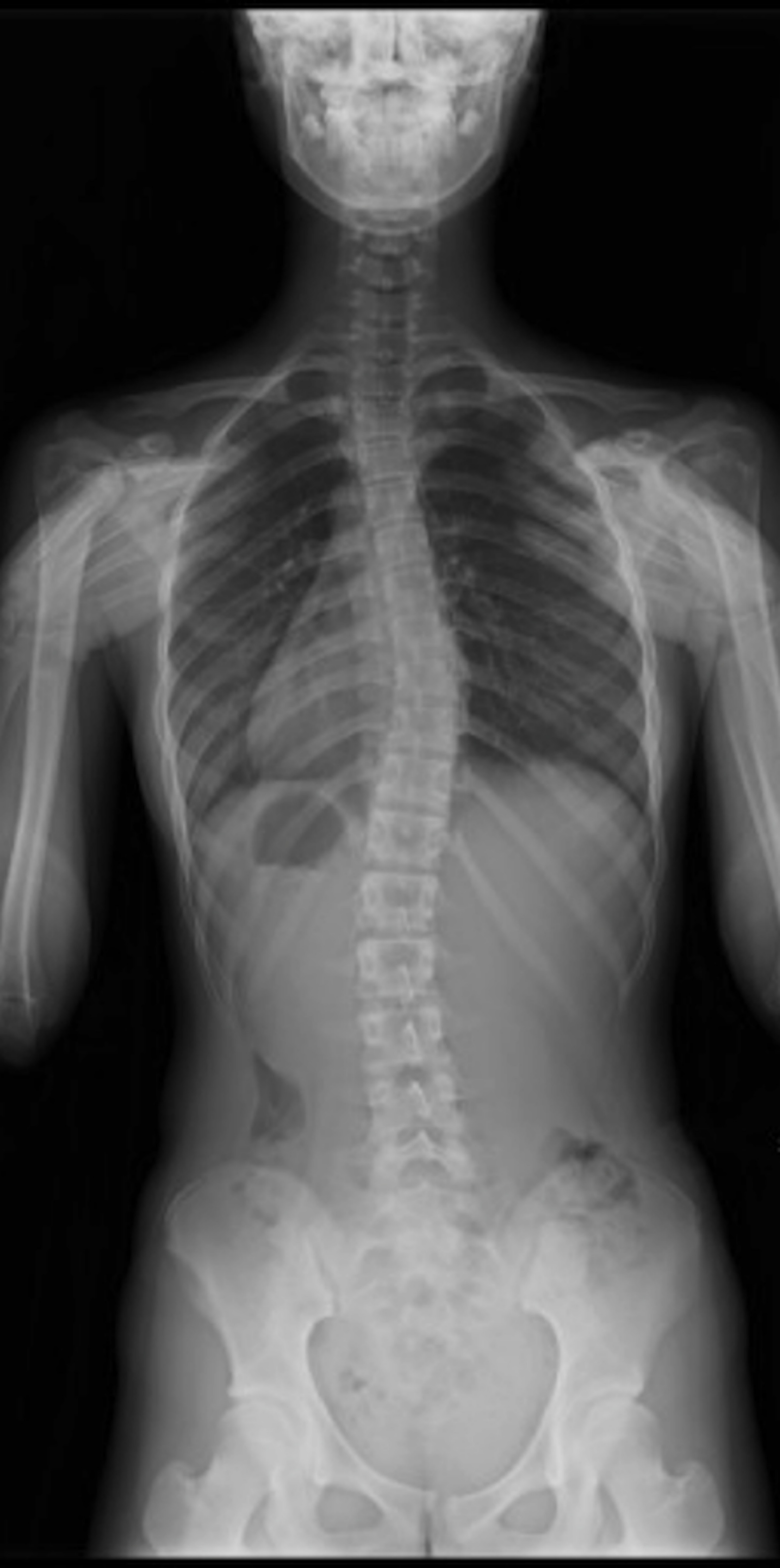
Standing posteroanterior radiograph (whole spine) of the same subject Cobb angle 16° for T5-T11
